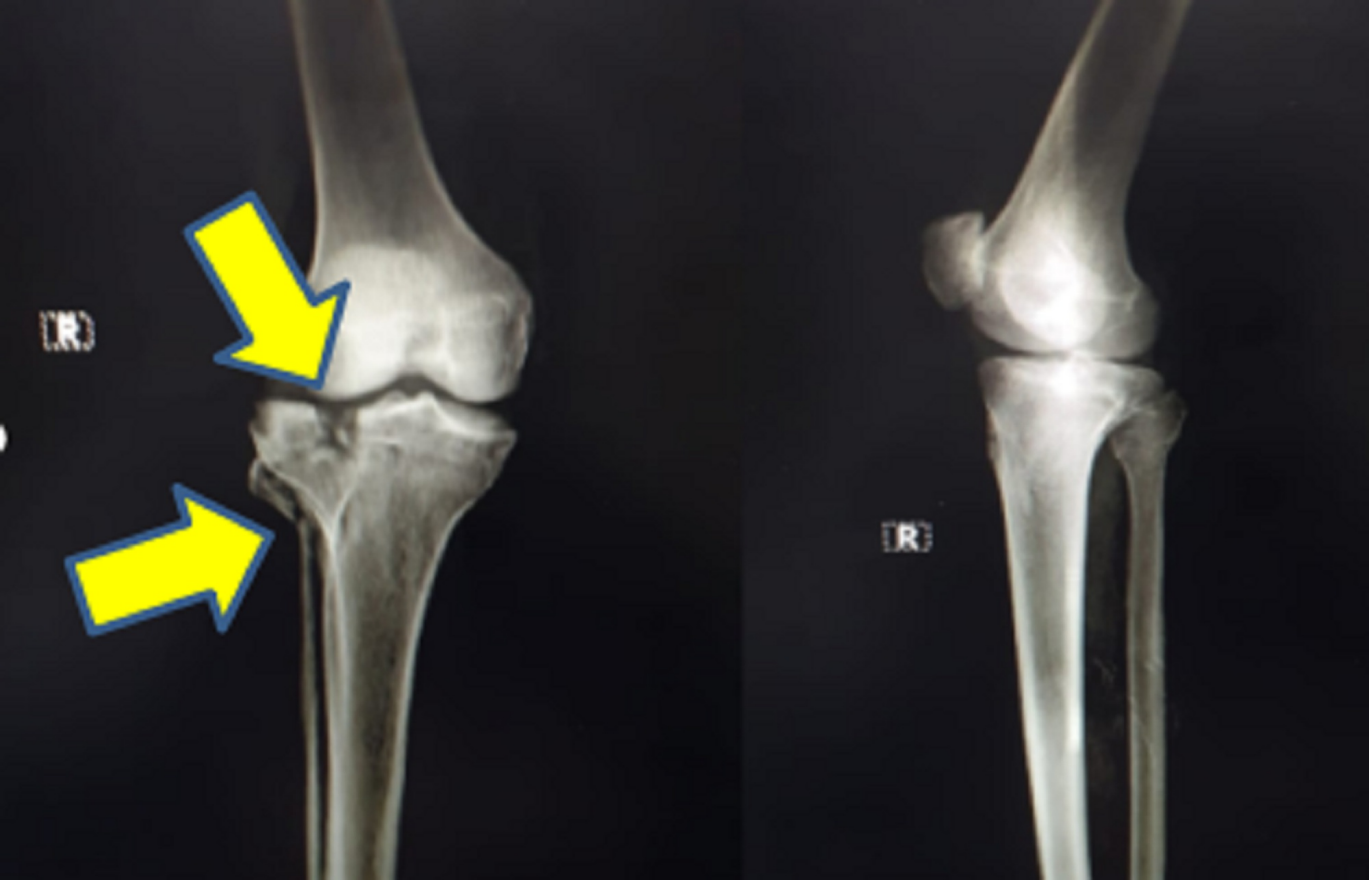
Plain radiograph (anteroposterior and lateral view) of the right knee showing split fracture involving the lateral tibial condyle, extending into the articular surface, and oblique fracture of the fibular head.
